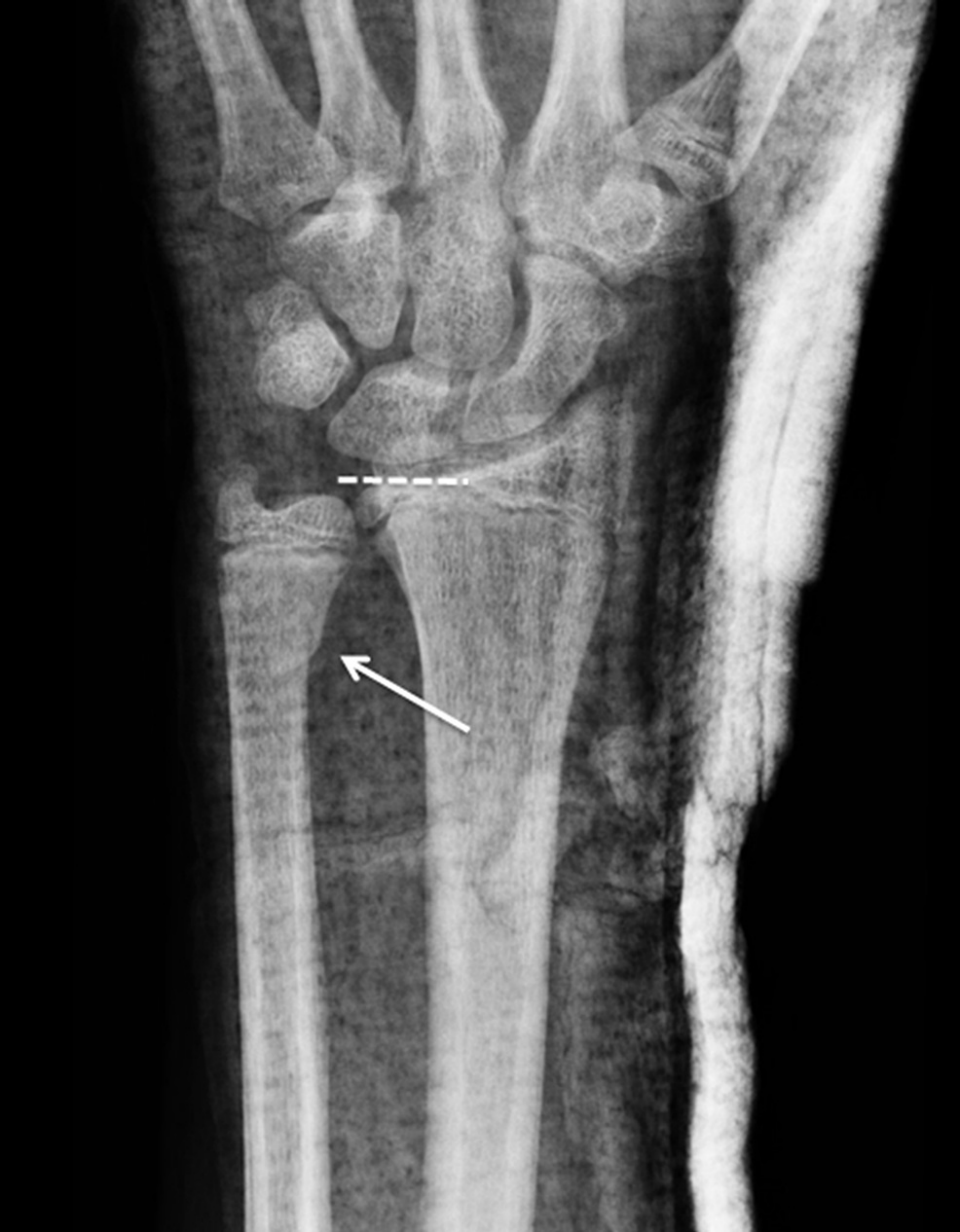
Anteroposterior radiograph of the left wrist following reduction.
